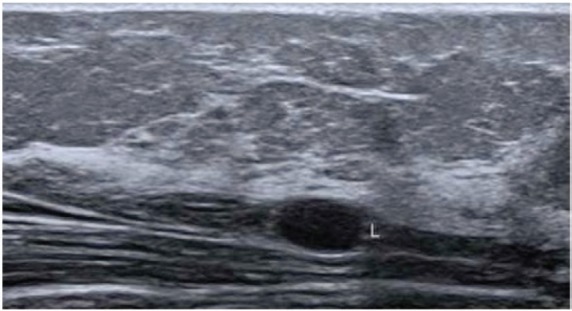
Ultrasonography showing subcutaneous fluid collection in the anterior aspect of the left arm root.
